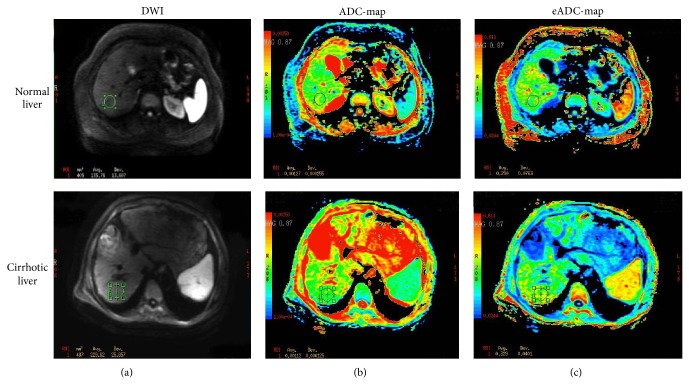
MR-DWI in a normal and cirrhotic liver (*b* value 600 s/mm^2^). DWI images are shown in the far left column. The middle column shows ADC maps superimposed on the corresponding anatomic images. The exponential ADC maps are shown in the far right column. The ADC maps show that the mean ADC value of the cirrhotic liver is lower than that of the normal liver (1.12 × 10^−3^ mm^2^/s versus 1. 27 × 10^−3^ mm^2^/s, resp.).
